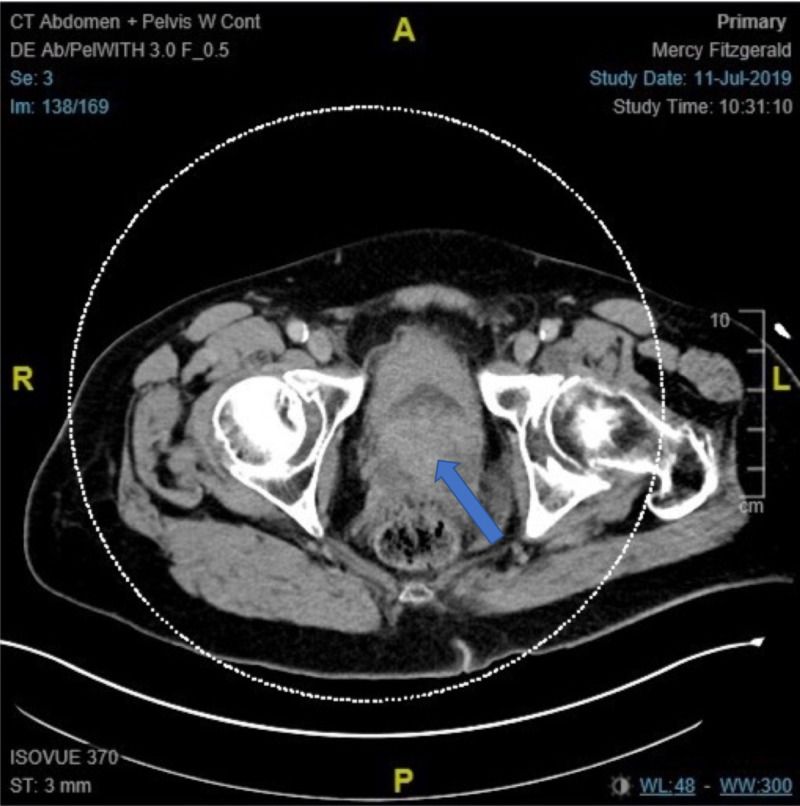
Computed tomography scan of abdomen and pelvis showing tumor of urinary bladder with involvement of prostate gland (arrow).
